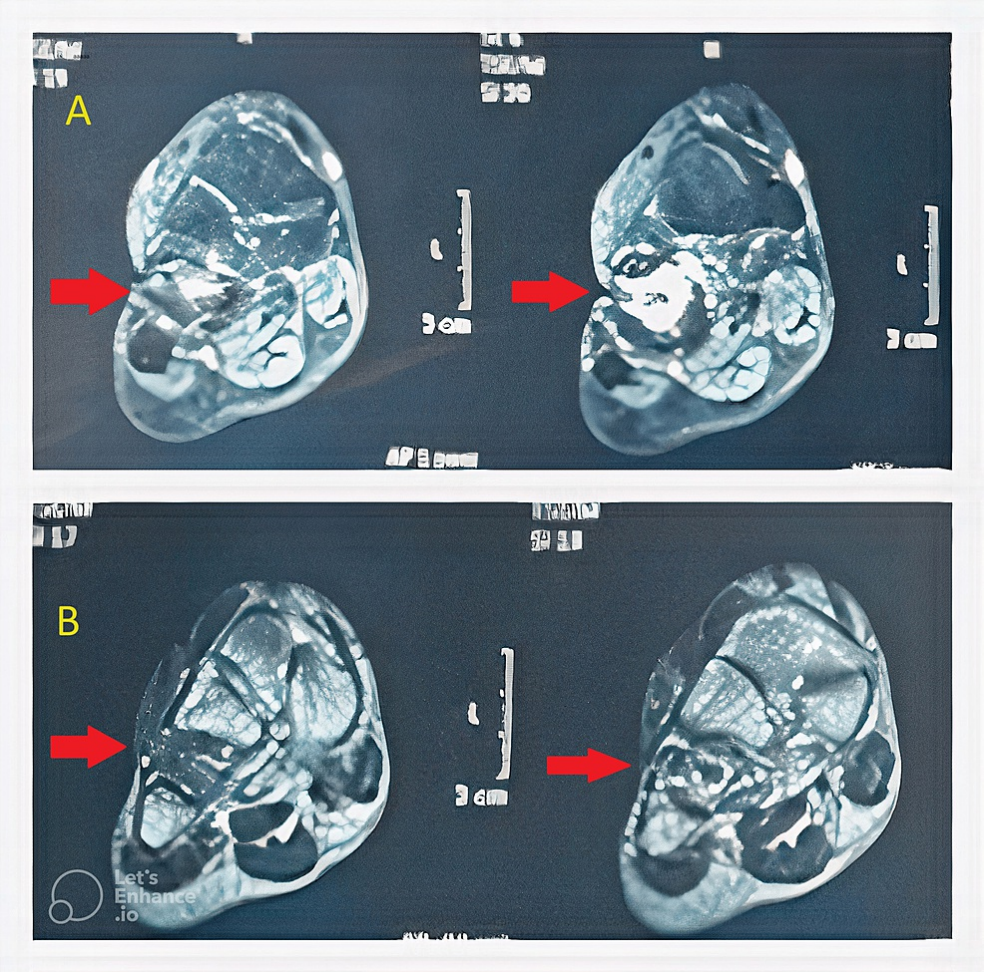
MRI of the right foot T2W (A) and T1W (B) images in the coronal view showing T1W hypointense signals and T2W hyperintense marrow signals involving the cuboid bone with an associated cortical break and sinus tract suggestive of osteomyelitis
